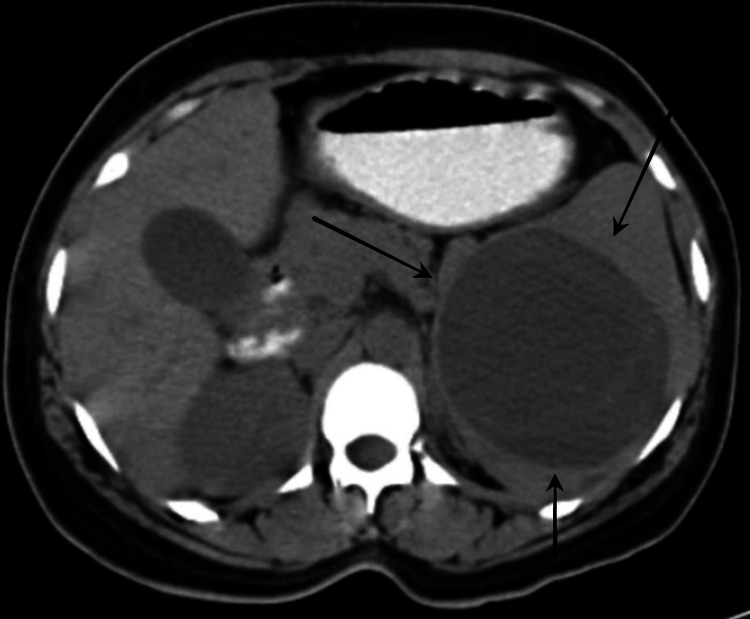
Contrast-enhanced computed tomography of the abdomen revealing a cystic lesion in the spleen (black arrows)
